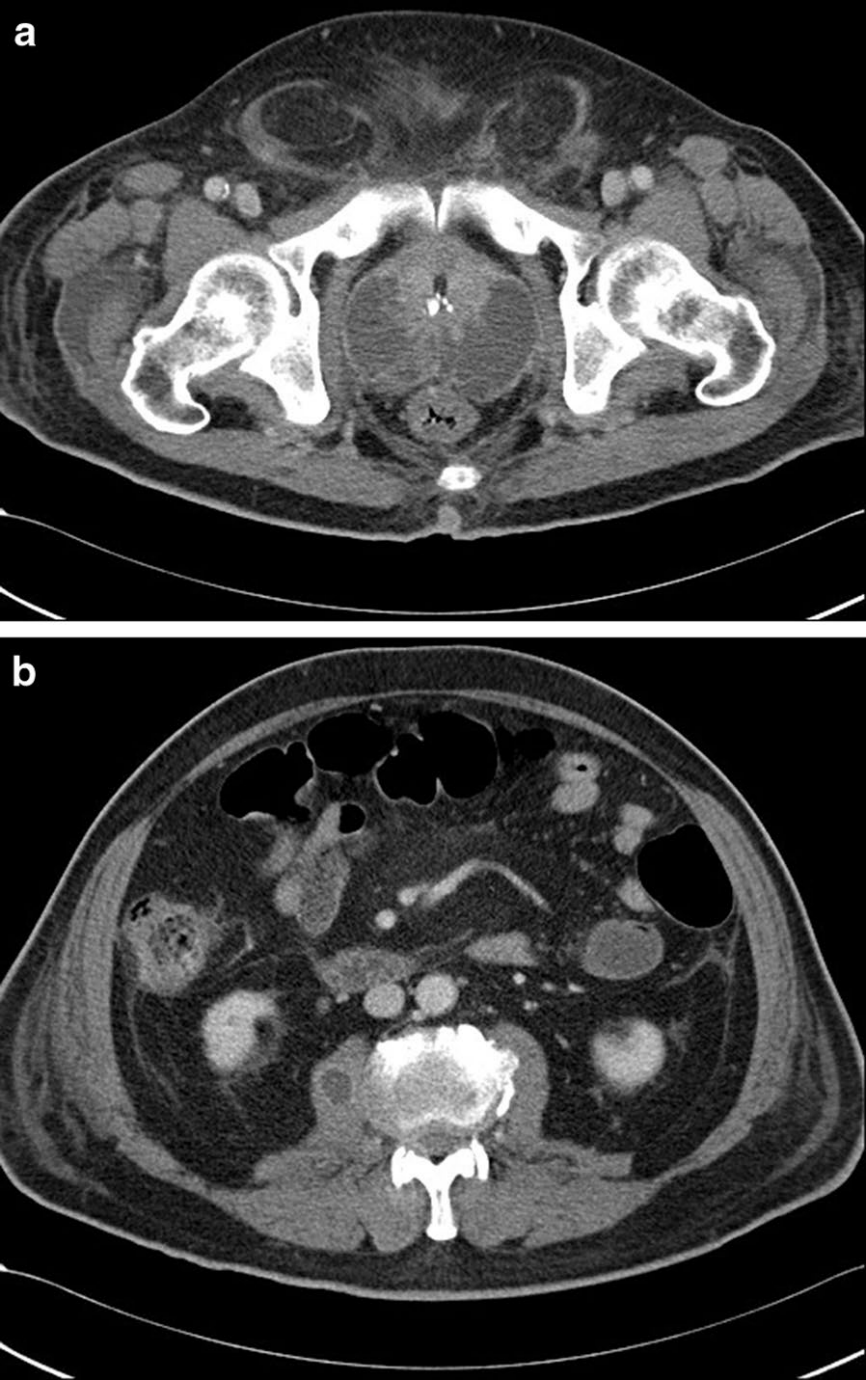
a Contrast enhanced abdominal CT depicted prostate abscess with calcification noted in the urethral wall. b Contrast enhanced abdominal CT dipicated right psoas muscle abscess
